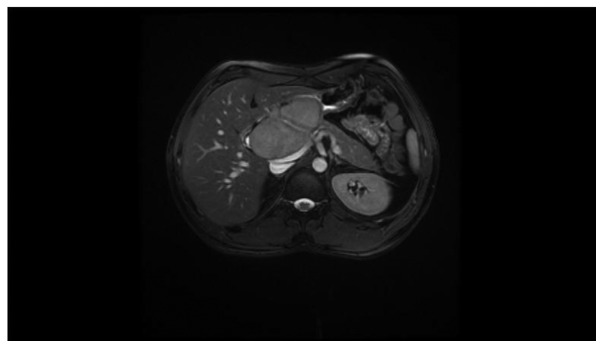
Axial gadolinium-enhanced T1-weighted fat-suppressed magnetic resonance image shows significant enhancement of the mass at the pancreas head and uncinate process.
